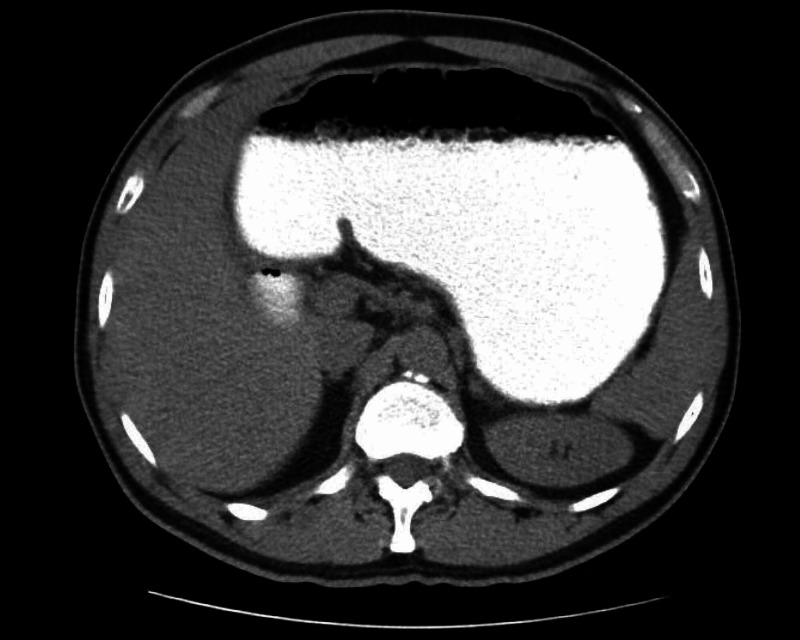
CT scan. CT scan after gastrografin administration revealing distended stomach.
